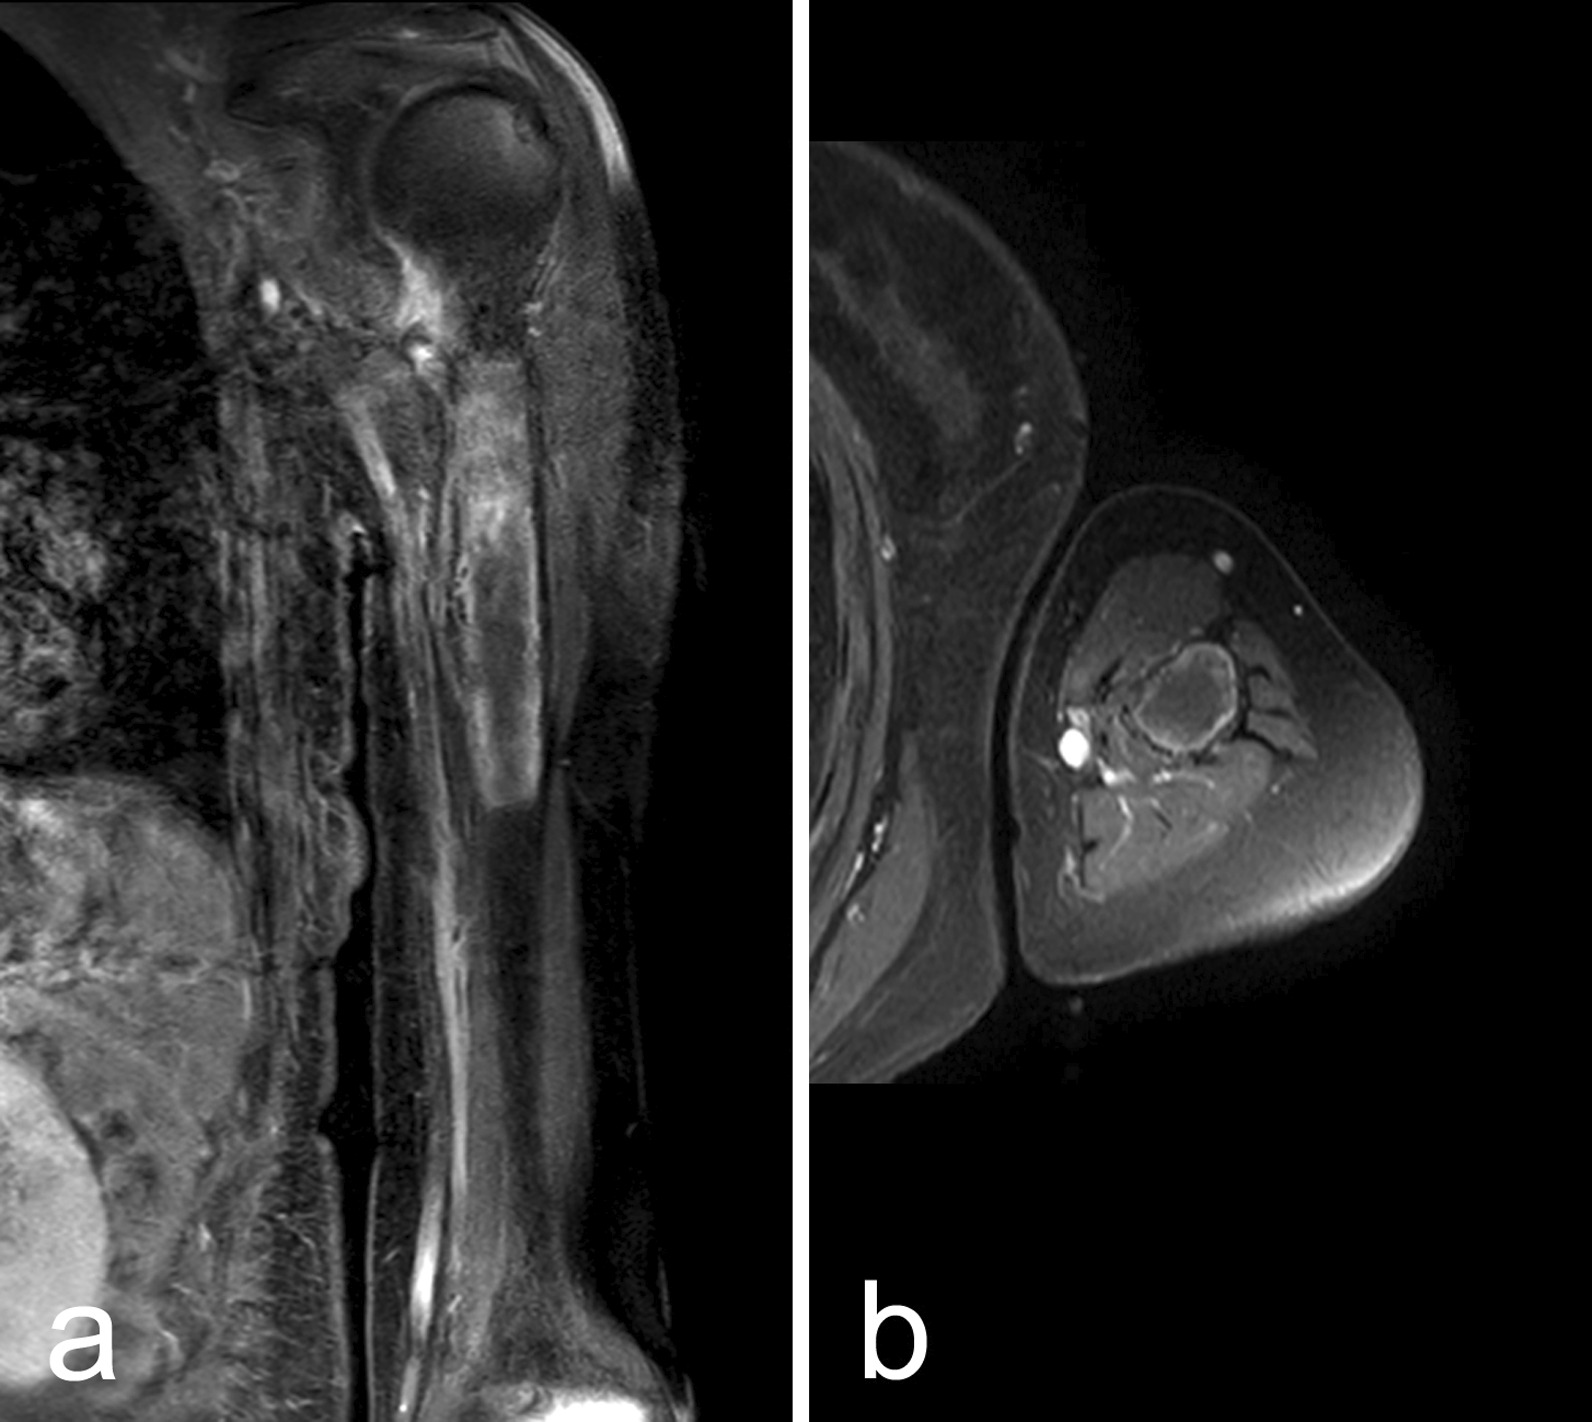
February 2019: MRI of the left humerus after 3 months imatinib treatment. Coronal (a) and axial (b) contrast-enhanced fat-suppressed T1-weighted images. See the central necrosis and residual peripheral enhancement
